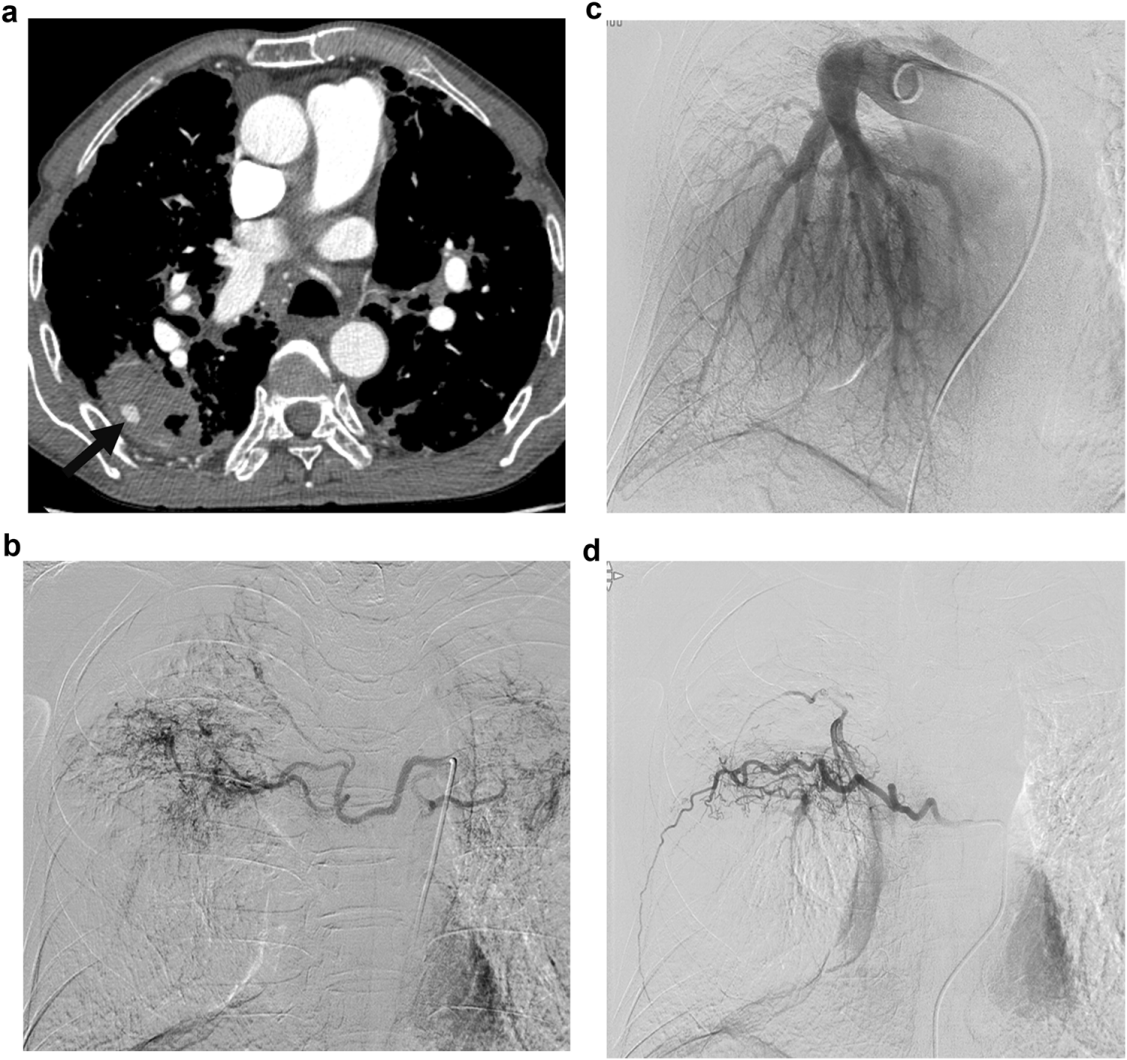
A 77-year-old male was admitted with hemoptysis. a CT scan shows a pulmonary artery pseudoaneurysm (PAP) in the right lower lobe. The PAP is located in the tuberculous cavity, surrounded by hematoma, and isolated from the pulmonary arterial branch (arrow). b Pulmonary arteriography was performed using a pigtail catheter; however, the PAP could not be visualized. c, d The PAP was not visualized upon performing bronchial and intercostal arteriography. Empirical bronchial/non-bronchial systemic artery embolization (BAE/SAE) in the area corresponding to the PAP was performed using 355–500 microns PVA particles and hemoptysis stopped after embolization
